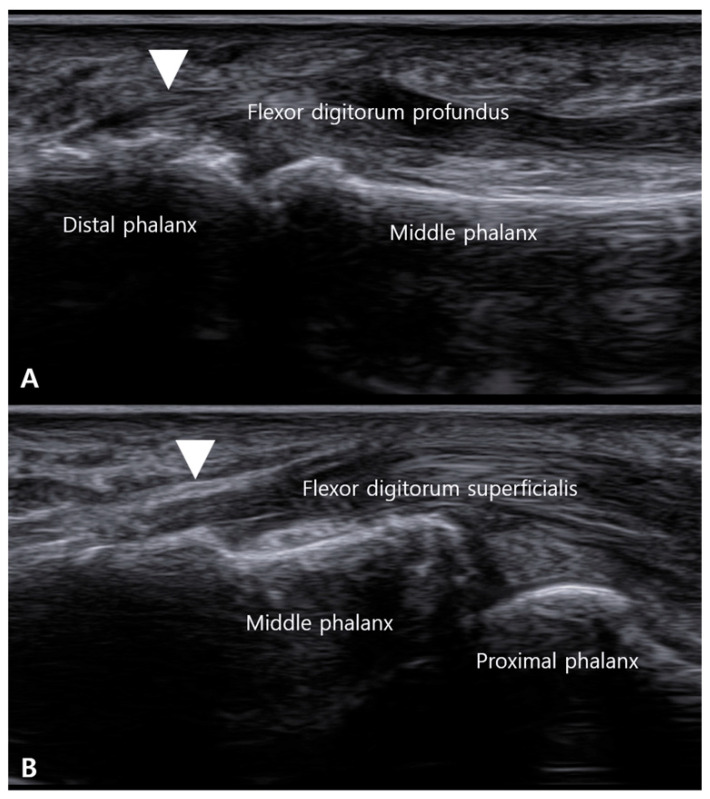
Sonographic evidence of insertional tendinopathy in the tendon-dominant phenotype. Long-axis ultrasound views demonstrate enthesopathic changes at the flexor tendon insertions. (A) At the distal phalanx, a palmar bony ridge (white arrowhead) is seen at the insertion of the flexor digitorum profundus (FDP). (B) At the middle phalanx, a similar bony ridge (white arrowhead) is present at the insertion of the flexor digitorum superficialis (FDS). These findings represent potential distal drivers of triggering in the tendon-dominant phenotype.
